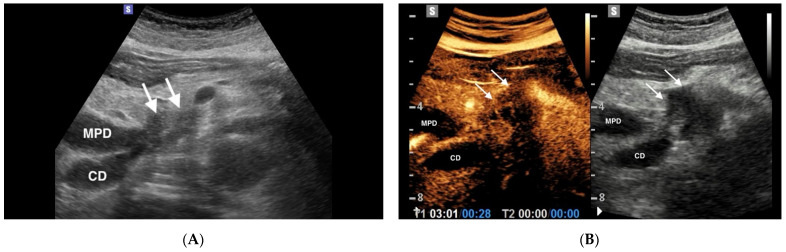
Pancreatic adenocarcinoma in an 89 years old woman. (A) B mode Ultrasonography depicts a 3.5 cm heterogenous hypoechoic mass (arrows) in the pancreatic head: severe dilation of the main pancreatic duct (MPD) and cystic duct (CD) is also appreciable. (B) On CEUS, in the arterial phase (28 s after the injection of c.a.) the mass is hypoechoic in comparison with the adjacent pancreatic parenchyma (arrows).
